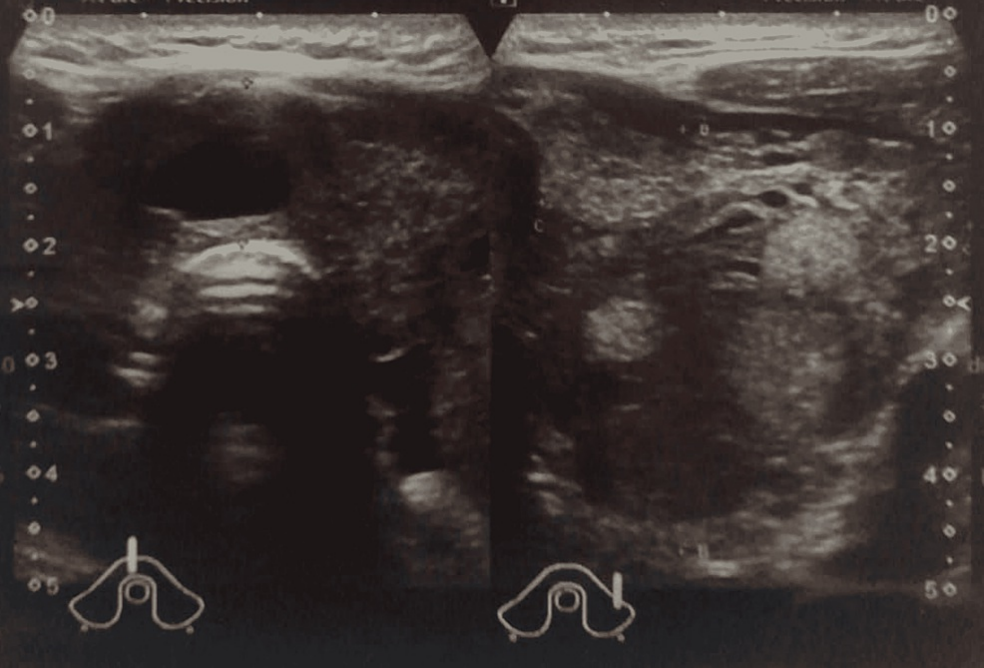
Patient’s thyroid ultrasound upon diagnosis showing multi-nodular goiter with the largest nodule measuring 24x22x21 mm in the lower pole of the left thyroid lobe.
